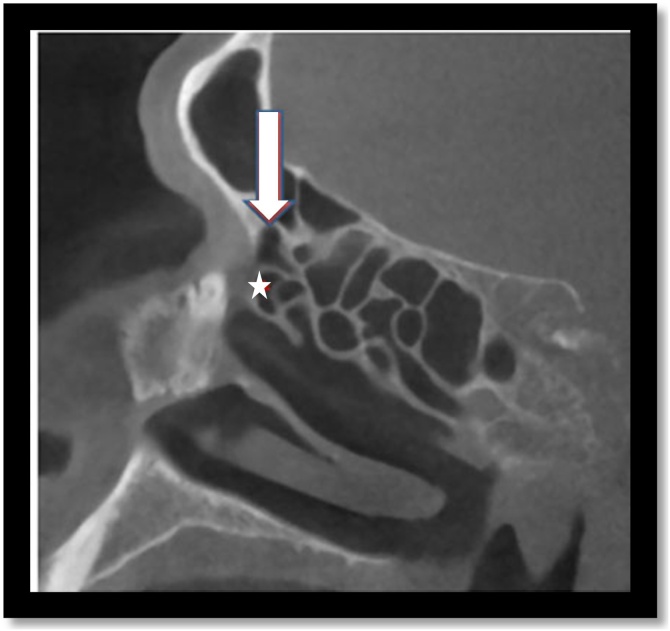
Supra-Agger cell. Sagittal computed tomography section demonstrates an example of Agger nasi cell (white star) and supra-Agger cell (white arrow).
